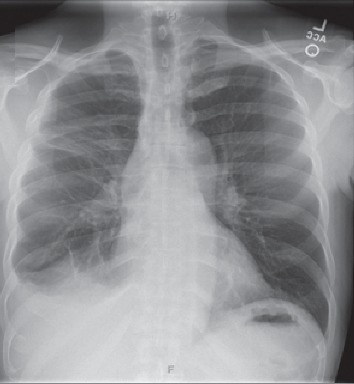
Postprocedure X-ray prior to discharge from the hospital showing improvement of bullae and expansion of the right lung.
